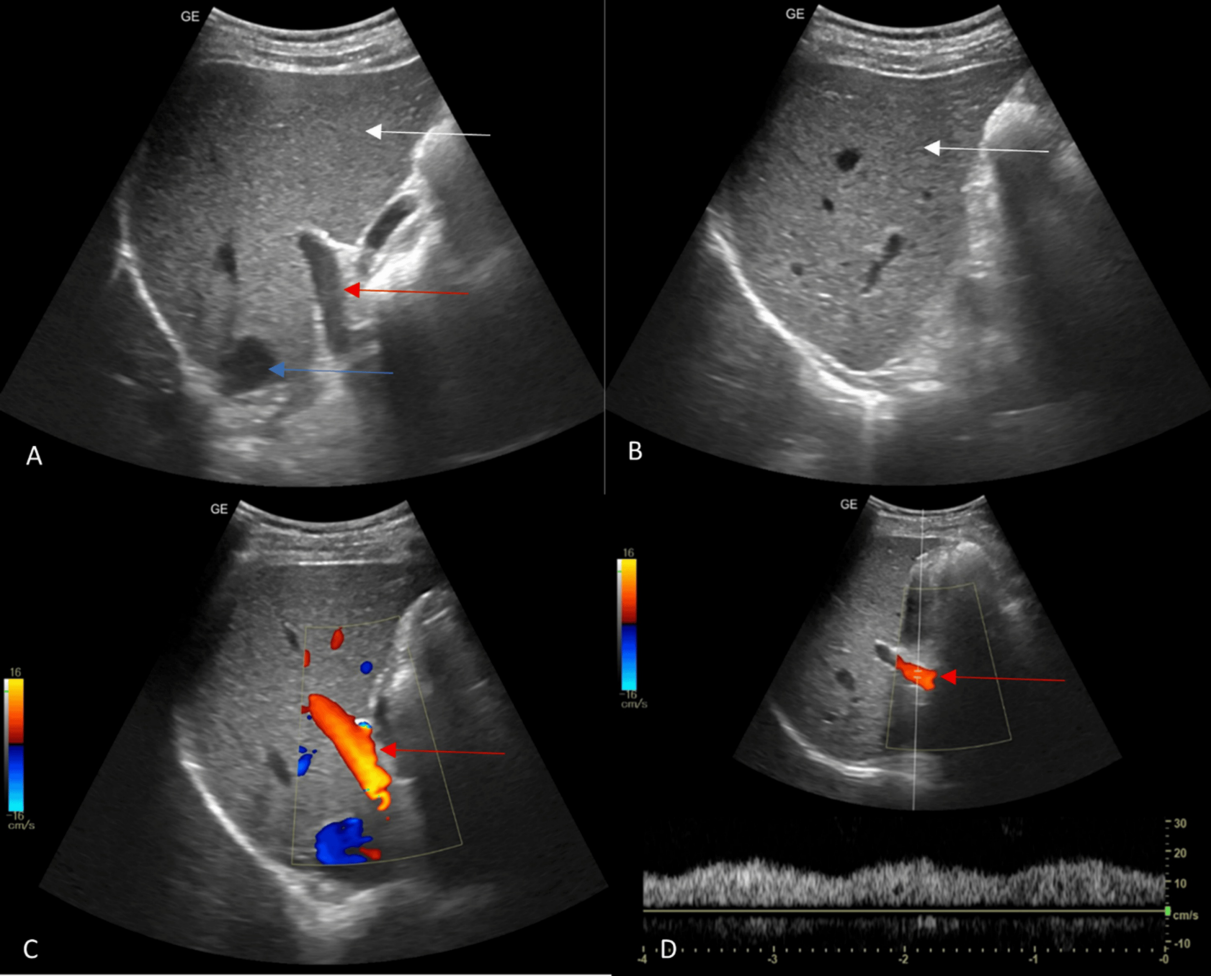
(A and B): Grey scale images of the liver show normal size, echotexture, and smooth surface of the liver (white arrow), normal diameter of the portal vein (red arrow) and IVC (blue arrow). (C and D) Colour and pulsed Doppler images of the portal vein show normal hepatopetal low (red arrows), normal velocity and waveform pattern. The images included in this review manuscript are from our own clinical cases and are used with appropriate permissions
